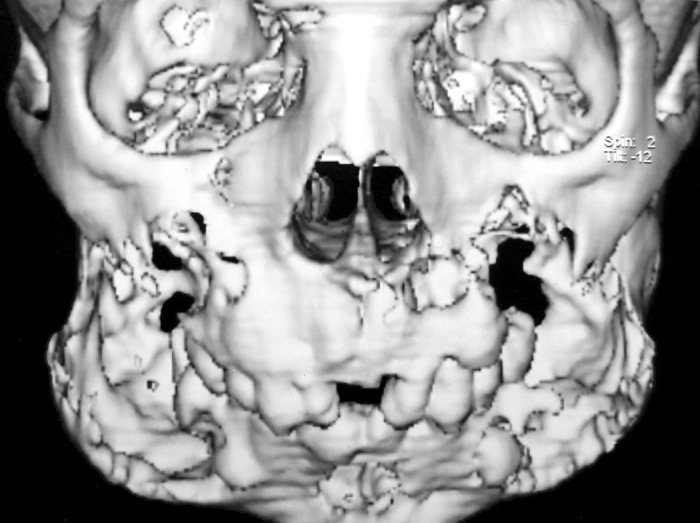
3D computed tomographic images showing osteolytic
